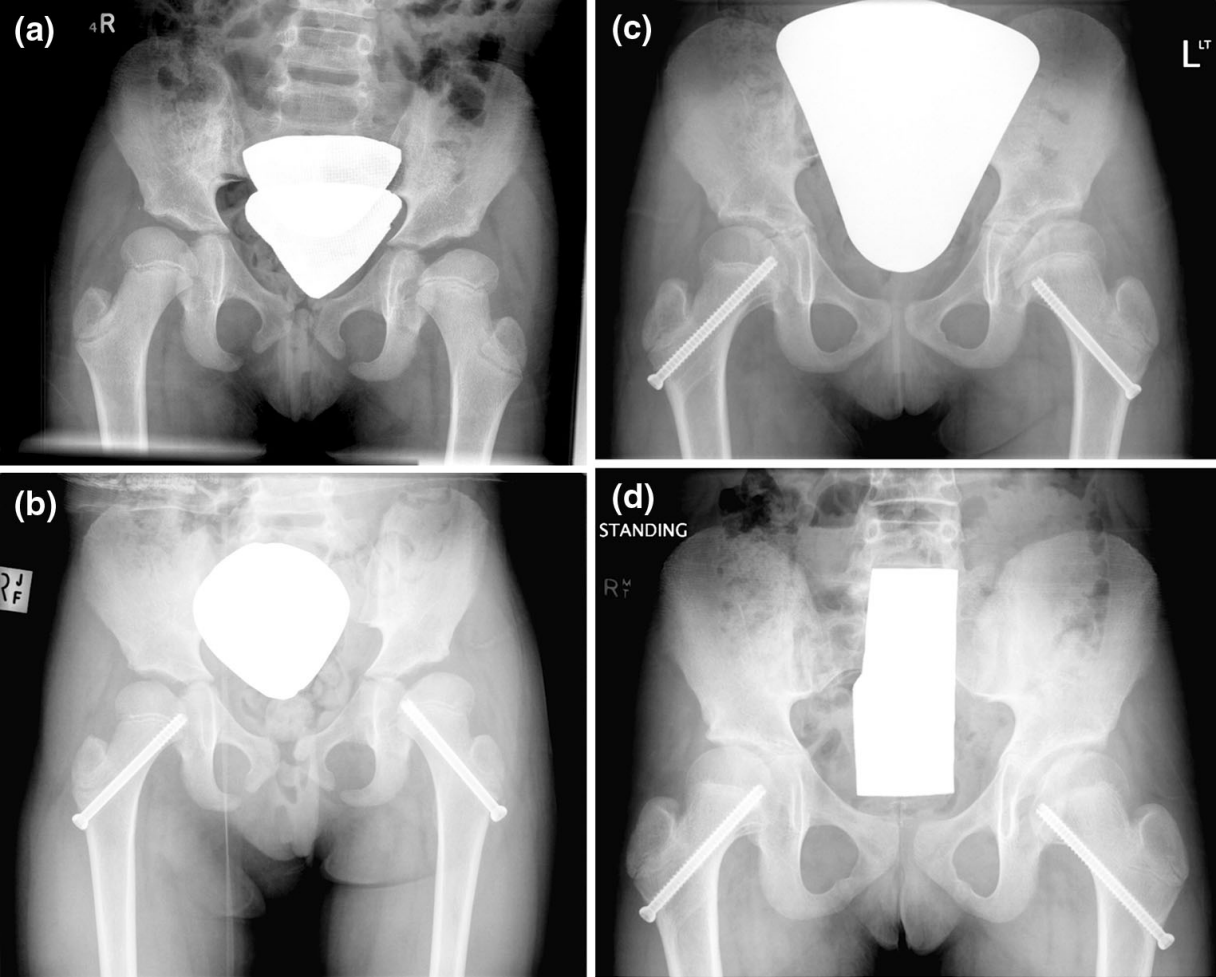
a Preoperative radiograph of a 7-year-old patient with bilateral DDH. b Pelvic radiograph following insertion of bilateral transphyseal screws. c Pelvic radiograph 4 years after insertion; the right transphyseal screw was revised and the left transphyseal screw requires revision. d Pelvic radiograph 5 years 6 months after the index insertion of bilateral transphyseal screws
